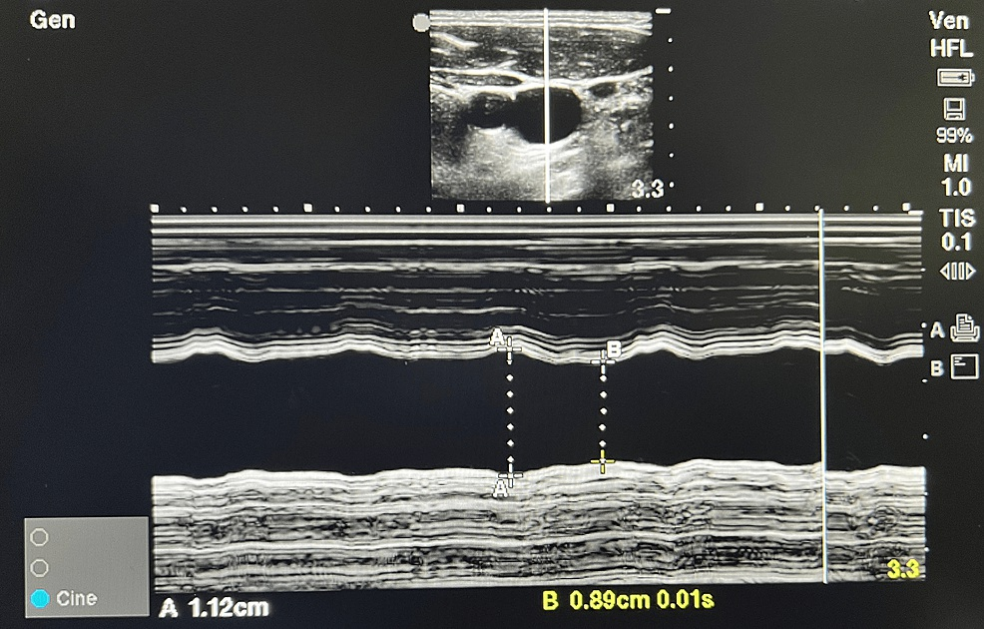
Ultrasound image of right IJV in M-mode IJV: Internal jugular vein A: End expiration and B: End inspiration
